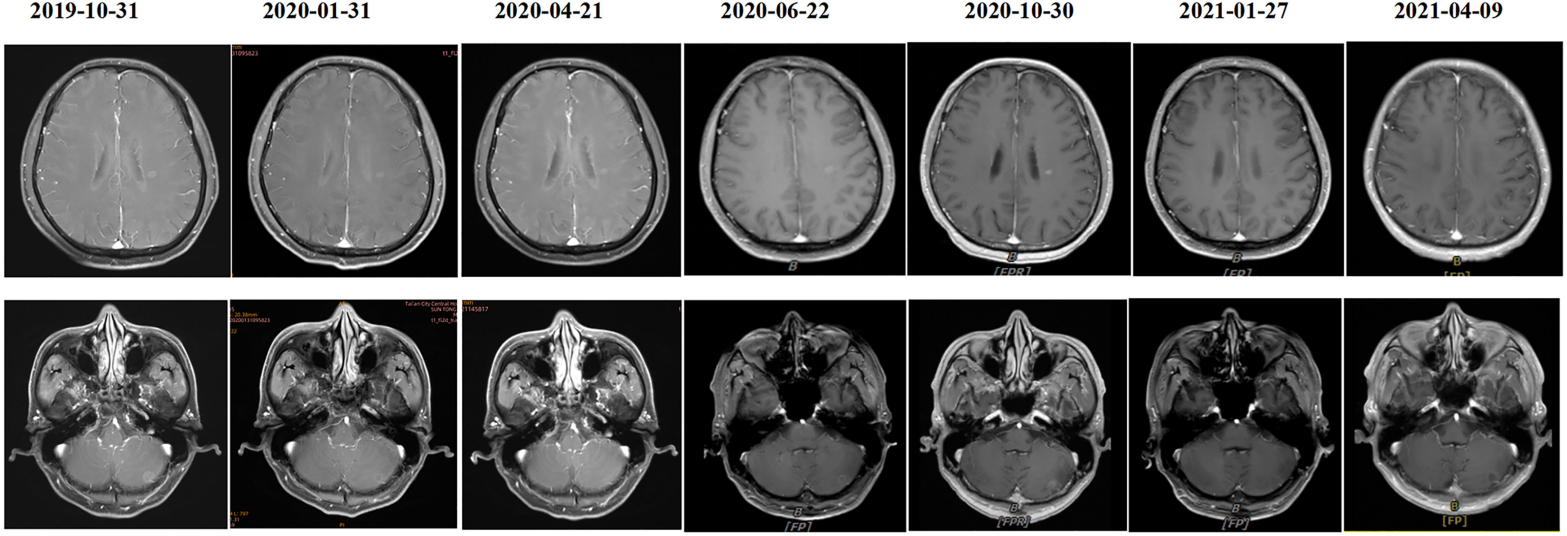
MRI imaging changes at different time points during the treatment process.
